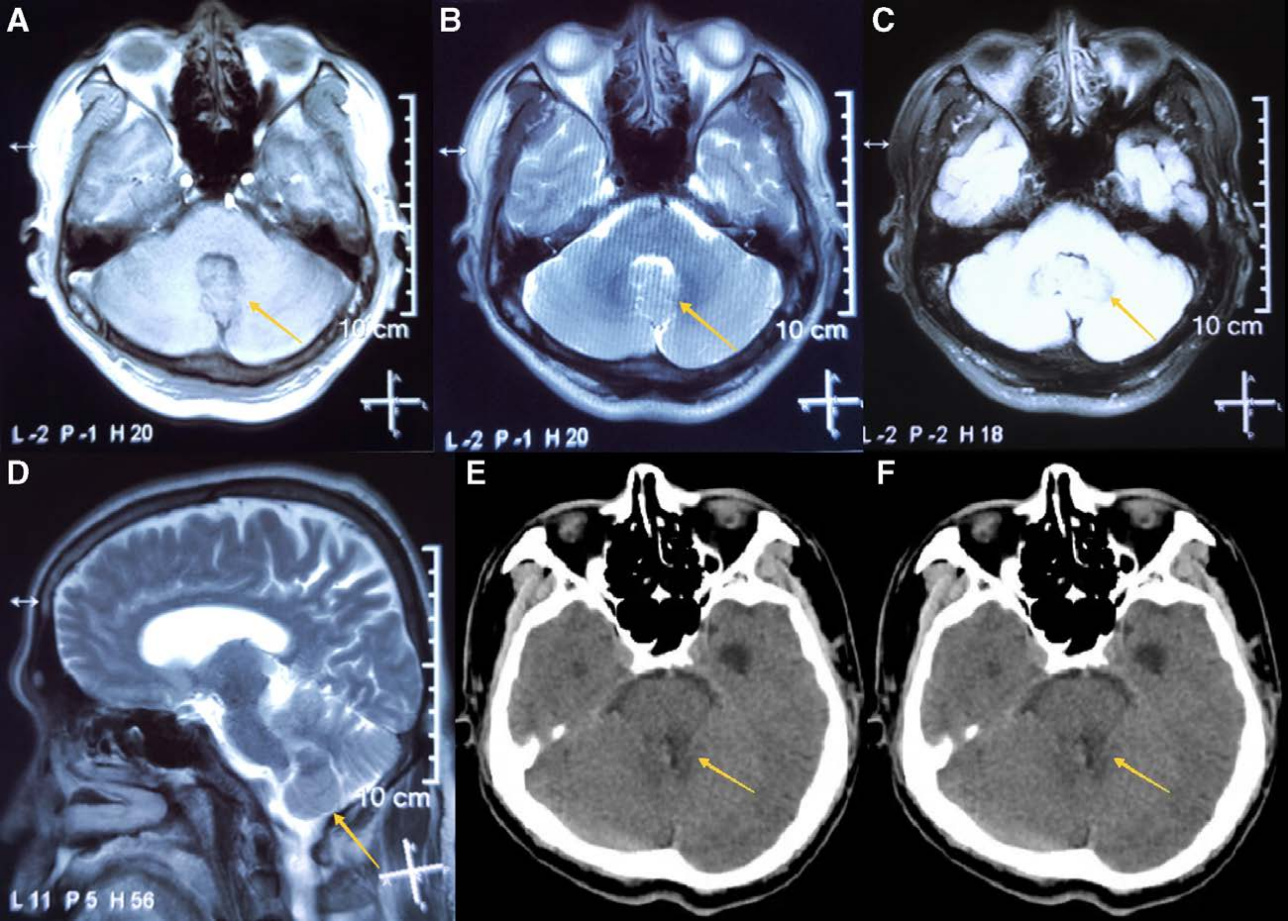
(A and B) MRI: T1-weighted and T2-weighted, images showing isointense and slightly hyperintense signals. (C and D) MRI: no obvious enhancement of the fourth ventricle lymphoma in the axial and coronal gadolinium-enhanced images. (E and F) CT: postoperative images (3 d). CT = computed tomography, MRI = magnetic resonance imaging.
